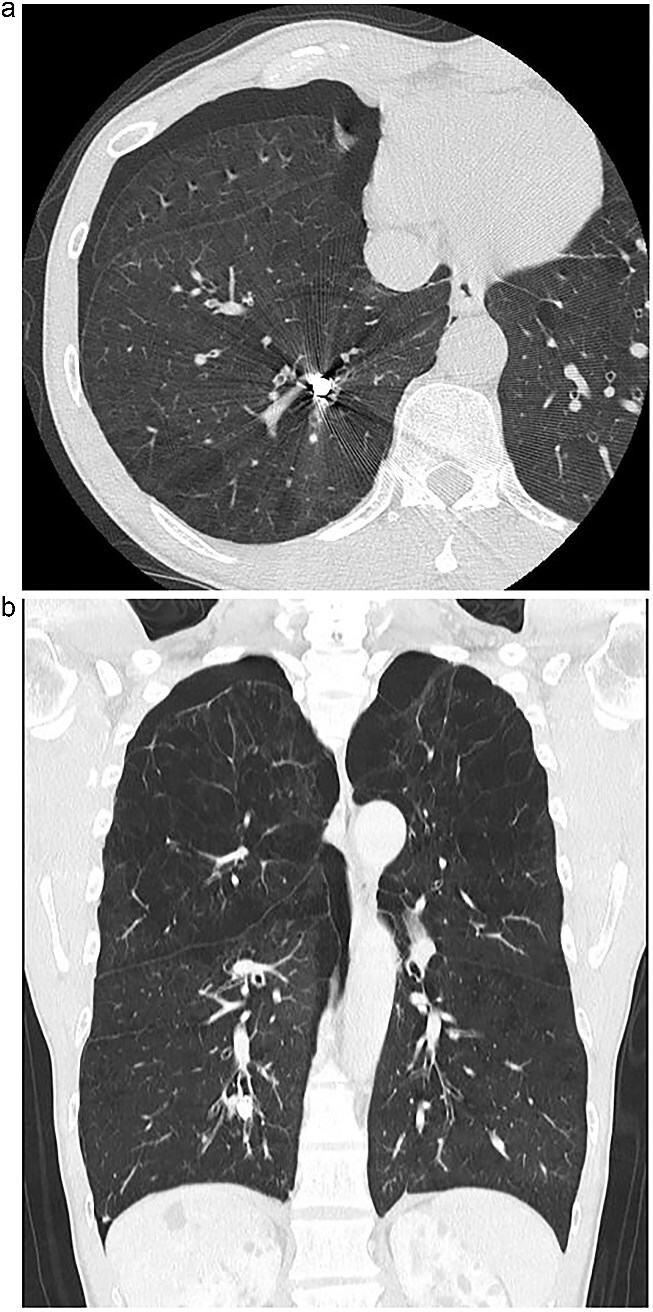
(a) Axial chest CT showing a high-density foreign body in the right S10 bronchus. (b) Coronal CT confirming the position of the dental prosthesis in the posterior basal segment.
